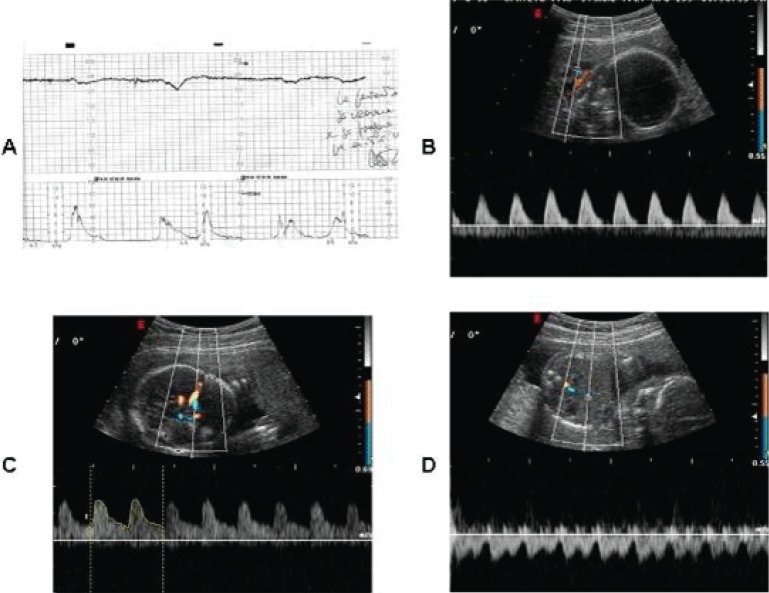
A. Fetal heart rate monitoring: decreased variability and tachycardia. B. Pulsed Doppler evaluation of the umbilical artery showing absence of the end-diastolic flow. C. Pulsed Doppler waveform of the ductus venosus at 39 weeks gestation showing reversed end-diastolic blood flow (during atrial contraction). D. Doppler sonography of the middle cerebral artery showed reduced resistance.
